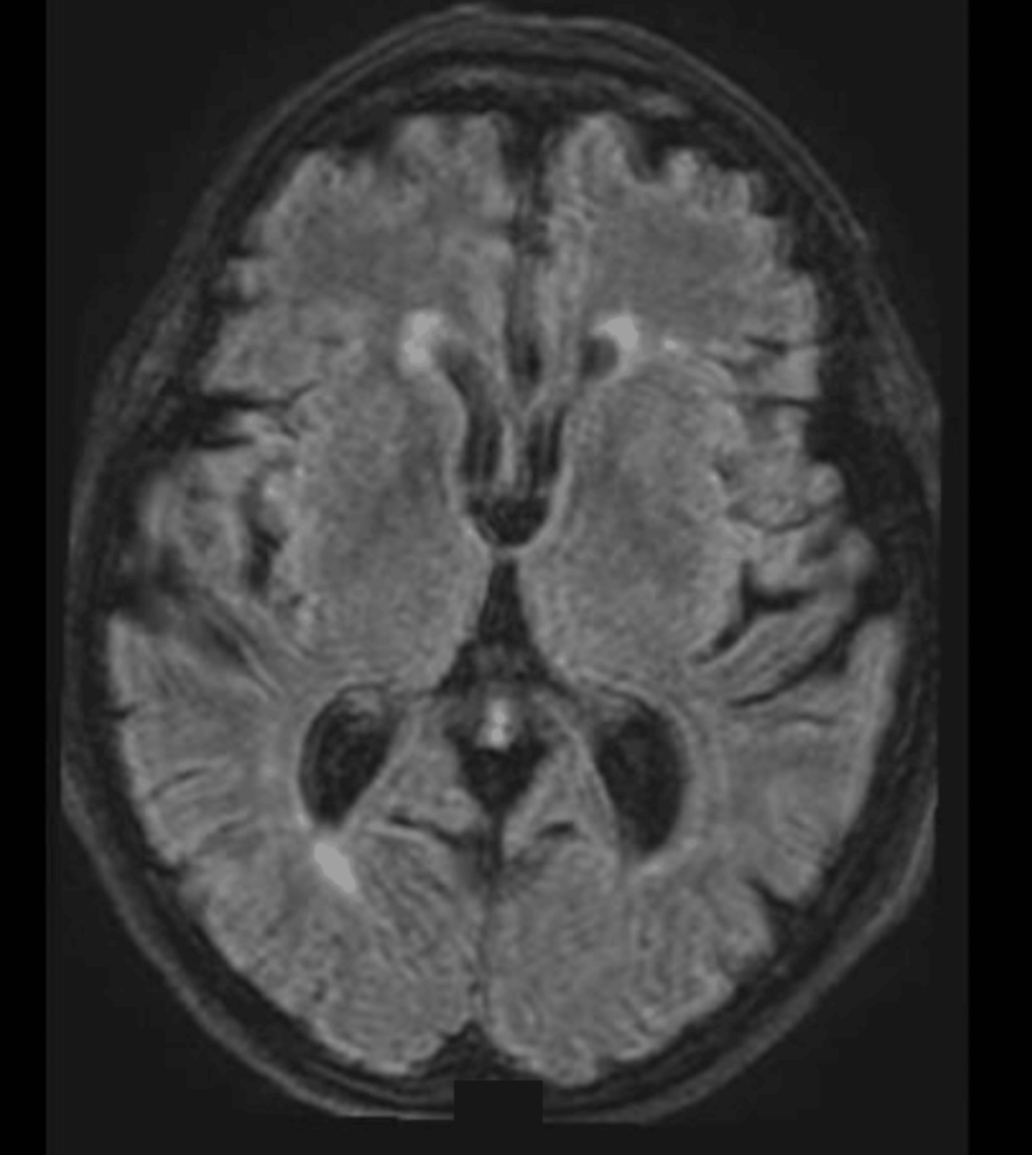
Brain magnetic resonance imaging Axial T2-FLAIR MRI sequence of the brain showing no focal abnormalities, structural lesions, or signal changes that could account for the patient's epileptic seizures. FLAIR: Fluid-attenuated inversion recovery.
